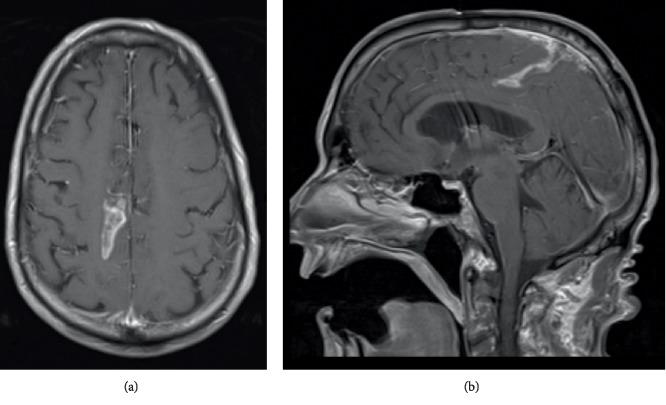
(a) Magnetic resonance imaging (MRI) T1-weighted axial image of the brain with gadolinium demonstrates leptomeningeal metastasis. (b) Corresponding sagittal image.
